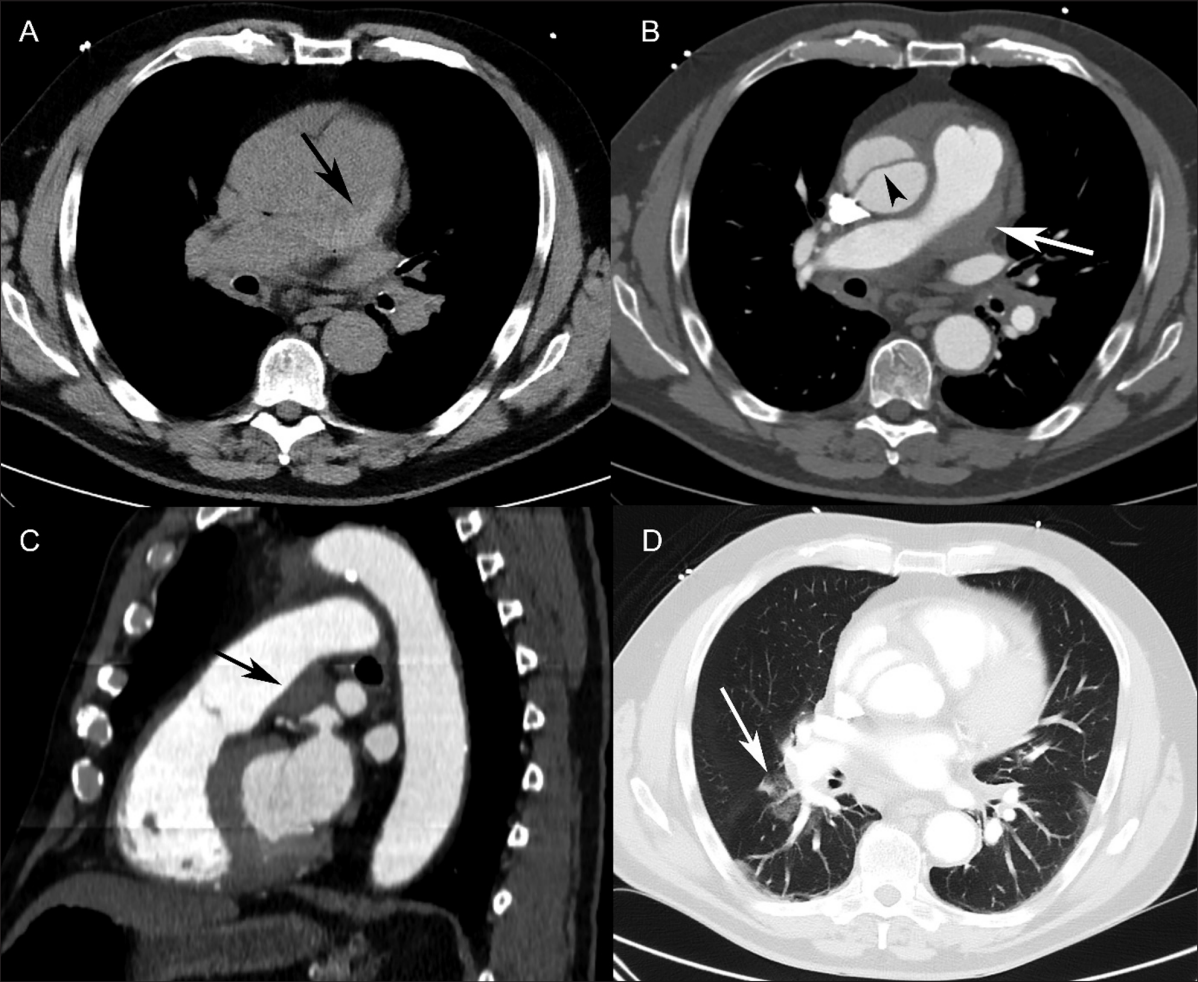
Patient 2. (A) Axial non-contrast-enhanced CT showed a hyperdense lesion along the pulmonary trunk wall (black arrow). (B) Contrast-enhanced CT (axial view) revealed an eccentric hypodense defect in the pulmonary trunk (white arrow), mimicking a mural thrombus. The intimal flap was seen in the ascending aorta (arrowhead). (C) Sagittal reformatting of a contrast-enhanced CT scan revealed a lesion with well-defined margins at an obtuse angle (black arrow) along the pulmonary trunk wall, resulting in a slight narrowing of the pulmonary trunk lumen. This was not enhancing, suggestive of an intramural hematoma. (D) Axial CT (lung window) showed patchy ground-glass opacities in the right lower lobe around the segmental pulmonary arteries, consistent with alveolar hemorrhage.
